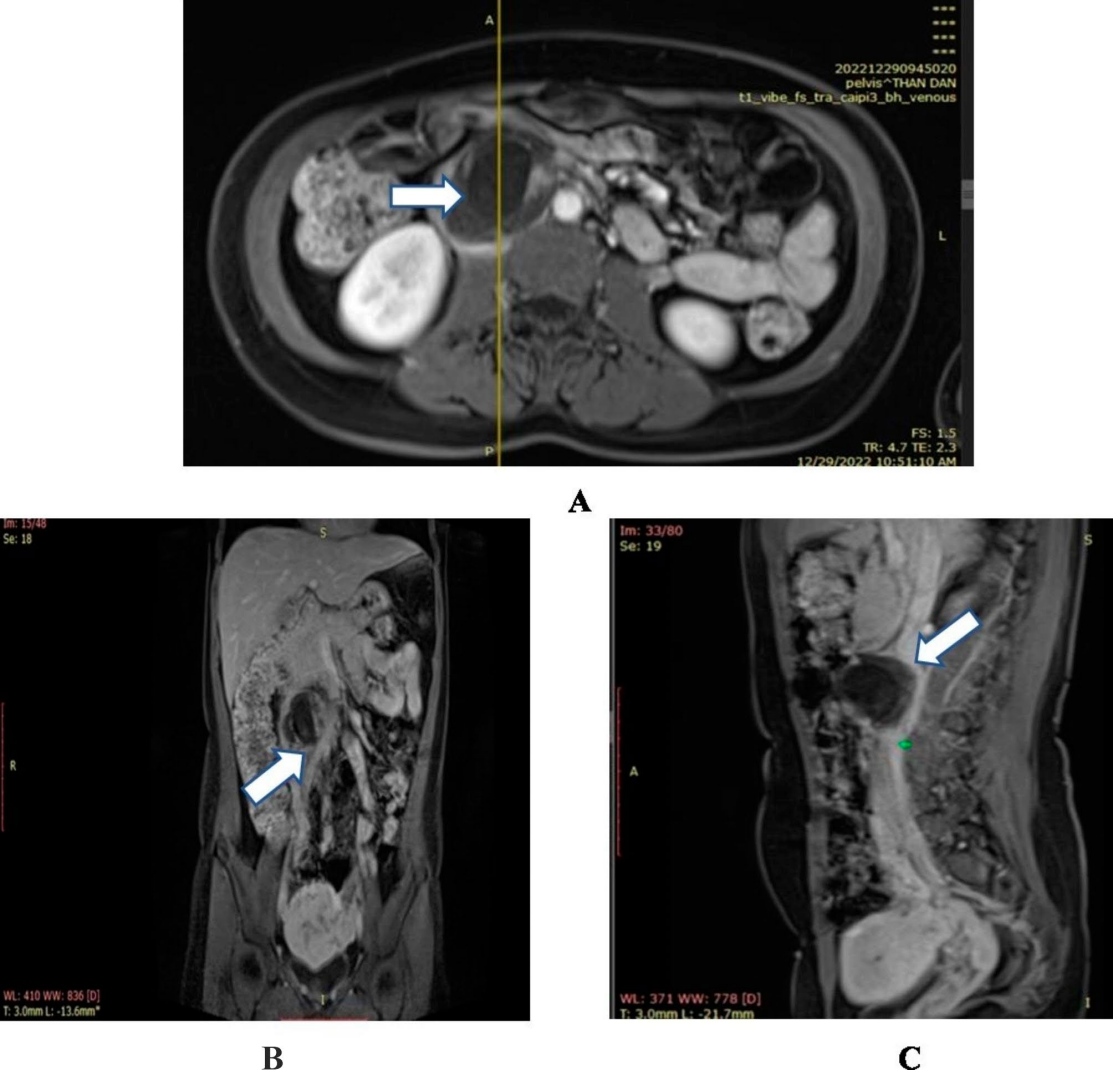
Magnetic resonance imaging in a 38-year-old female patient (G5P2) diagnosed a retroperitoneal ectopic pregnancy. Axial image showing a gestational sac measured 4.6cmx5.6cmx5.4 cm in size, invaded to the inferior vena cava in the retroperitoneal space, and tightly adherent to the abdominal aorta and duodenum (A). Coronal and sagittal images showed a gestational mass running from the lower pole of the right kidney, transverse to the vertebral column, and reaching to the bifurcation of the inferior mesenteric artery and two common iliac veins. The genital vein was dilated and in close contact with the gestational mass before attaching to the inferior vena cava. No fluid collection or hematoma was observed in the abdominal cavity (B-C).
